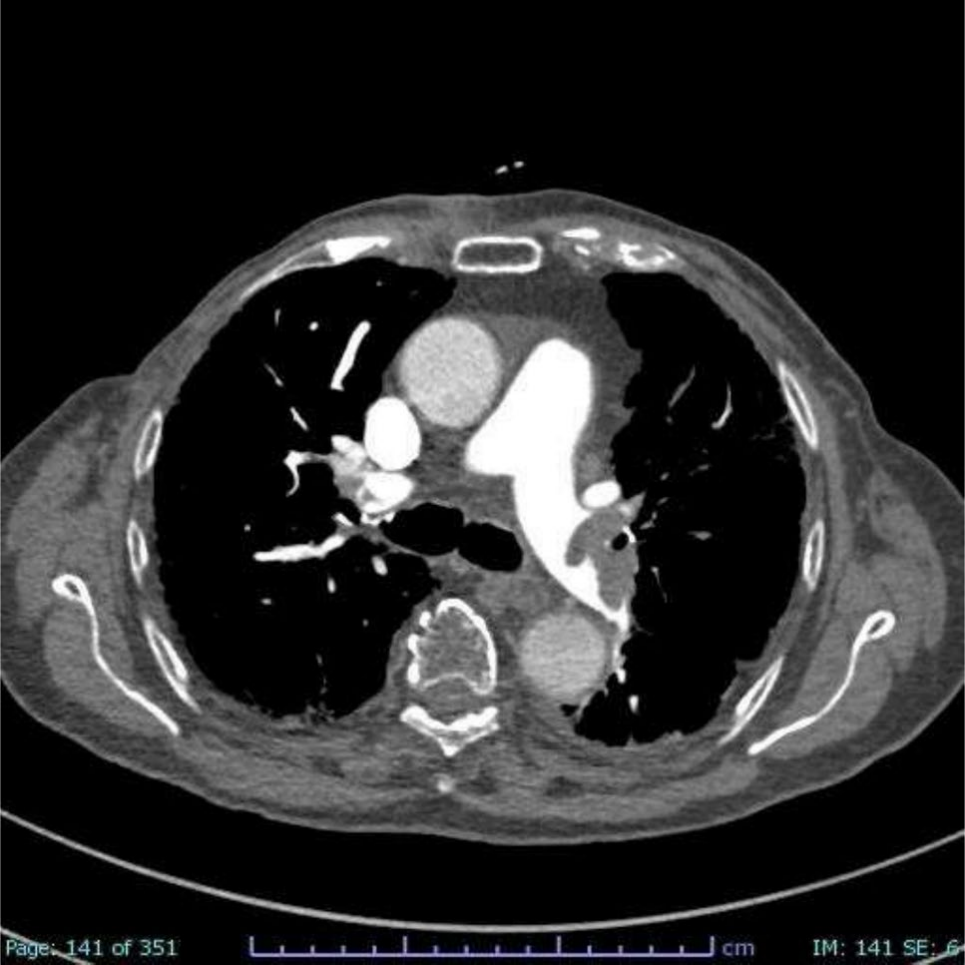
Axial CTA scan shows massive arterial occlusion with failure to enhance the entire lumen due to a substantial filling defect of both left and right lobar arteries
